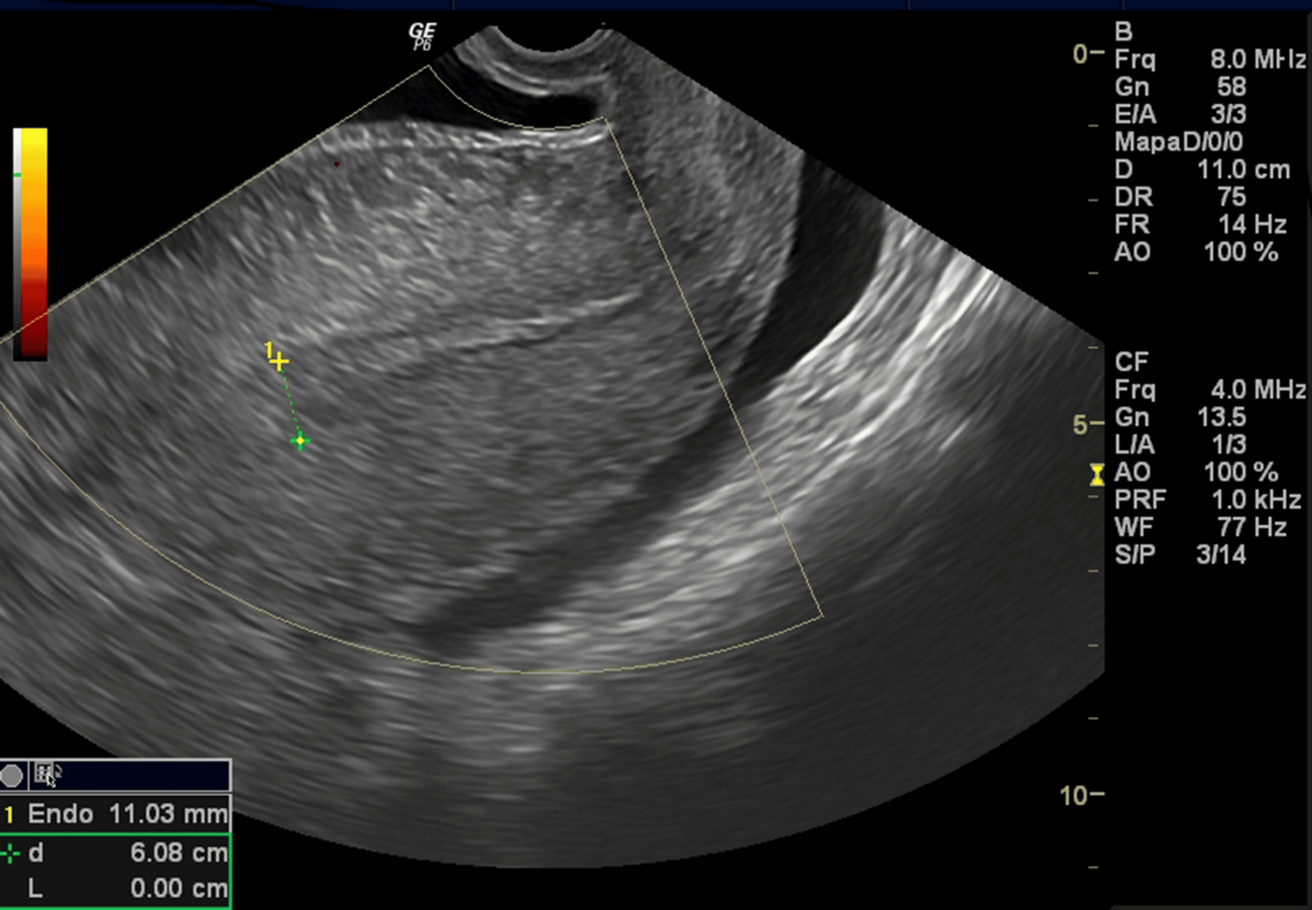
Uterus after evacuation. Transvaginal ultrasound showing the uterine cavity after evacuation, with residual heterogeneous endometrium measuring 11 mm by caliper.
